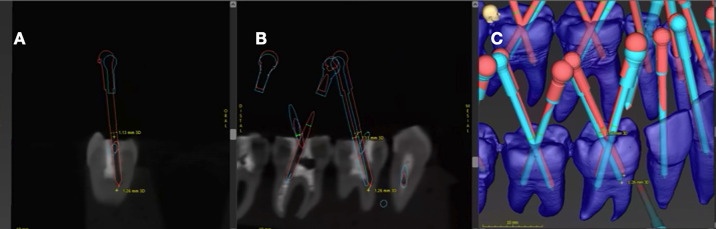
(A) Superposition of planned trajectories in a CBCT coronal section; (B) Sagittal section of measurement of CBCT deviations; (C) Volumetric reconstruction of trajectory deviation analyses.
